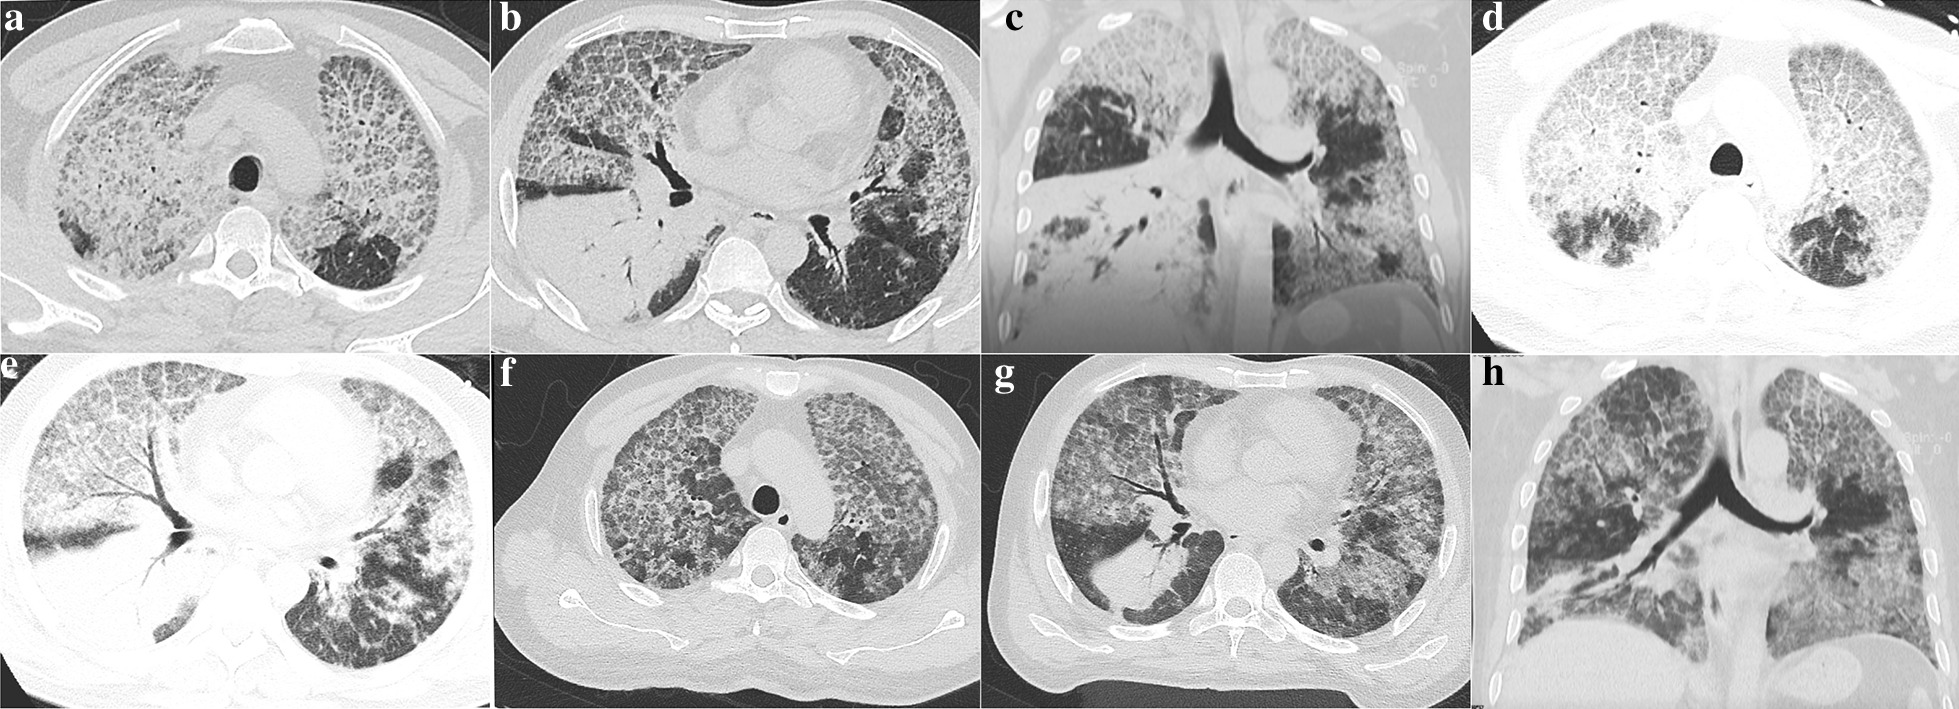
a–c (chest HRCT, June 23, 2020): bilateral, diffuse reticular pattern and ground-glass opacity (crazy-paving pattern) in the right upper and middle lobes and left upper lobe; exudation shadow in the left lower lobe; patchy consolidation in the right lower lobe. d, e (chest CT, July 13, 2020): exudation shadow in right upper and left upper lobes decreased; exudation shadow in right middle and left lower lobes increased; patchy consolidation in right lower lobe decreased slightly. f–h (chest HRCT, September 21, 2020): crazy-paving pattern in right upper and middle lobes and left upper lobe, and patchy consolidation in right lower lung both decreased significantly
